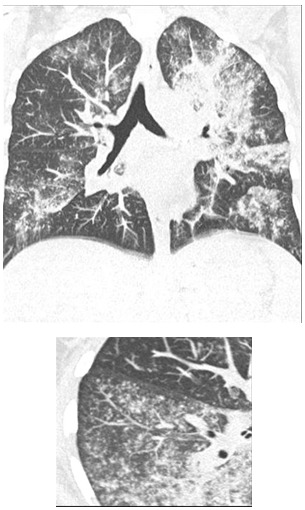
Volumetric reconstruction of the Thoracic computed tomography-scan at admission, showing multifocal areas of branched centrilobular nodules and patchy ground-glass opacities with few lobular reticulates (left). At right a single 5-mm thick slice of the upper right lobe.
